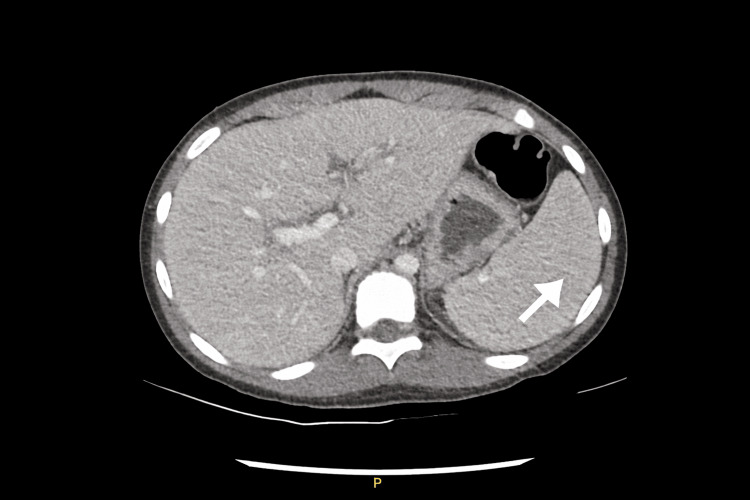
Axial contrast-enhanced CT image of the abdomen showing mild splenomegaly without focal splenic lesions
